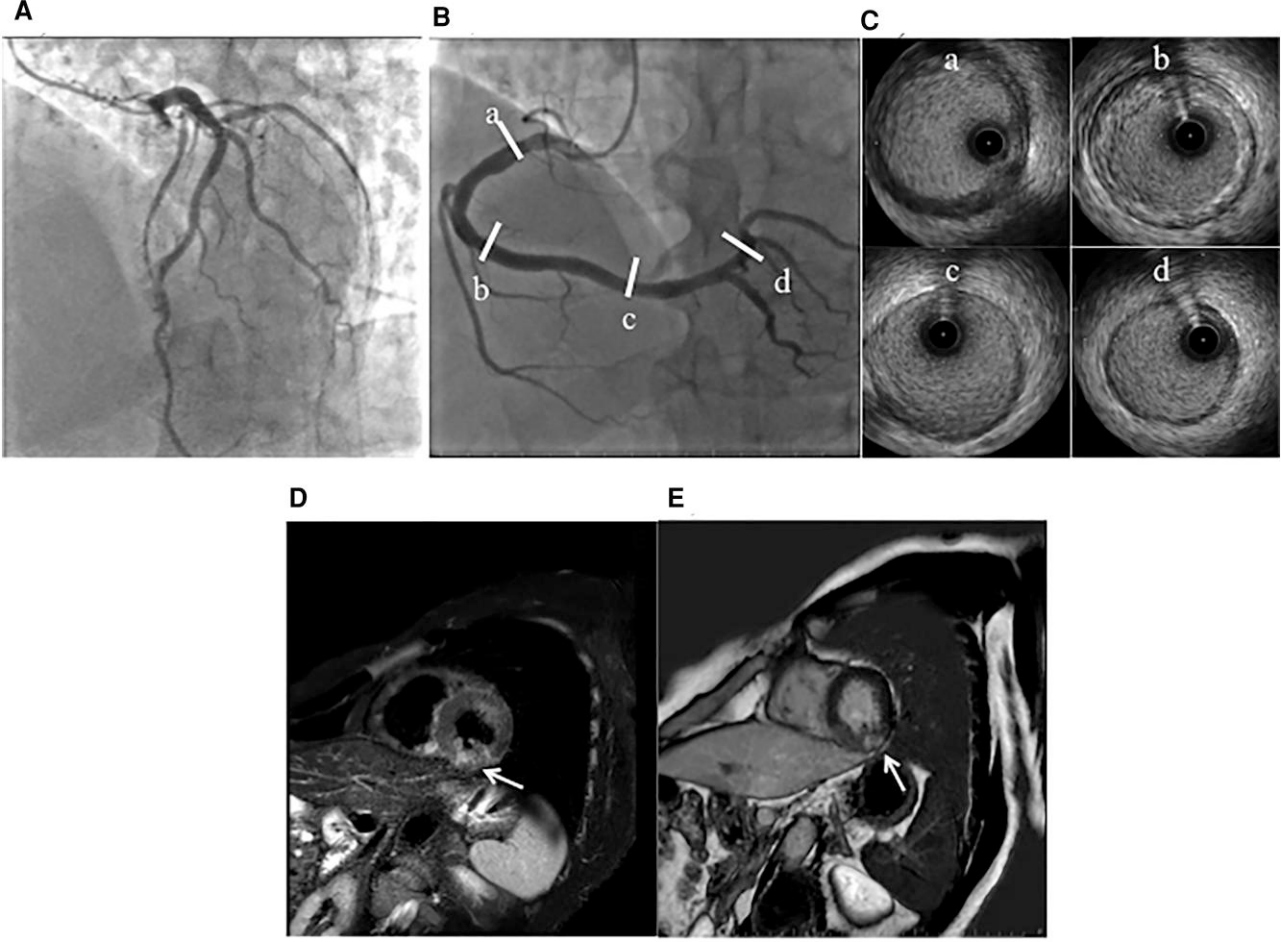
Coronary angiography and cardiovascular magnetic resonance imaging during the first hospitalization. There was no organic stenosis in the coronary artery after the intracoronary administration of isosorbide dinitrate (*A* and *B*). We subsequently performed intravascular ultrasonography of the right coronary artery, and evidence of thrombosis, plaque rupture, and coronary artery dissection was not observed (*C*). (*A*) Angiography of the left coronary artery from the LAO-cranial view. (*B*) Angiography of the right coronary artery from the LAO-cranial view. (*C*) Intravascular ultrasonography of the right coronary artery. The marks a, b, c, and d represent the intravascular ultrasonography position of the right coronary artery, as shown (*B*). In the short-axis cardiovascular magnetic resonance images, increased signal intensities in the T2-weighted short *τ* inversion recovery black-blood image (arrow) indicate recent myocardial oedema or inflammation (*D*). Late gadolinium enhancement imaging revealed irreversibly damaged myocardium in the same area (*E*, arrow). A diagnosis of acute or sub-acute inferior myocardial infarction was established.
